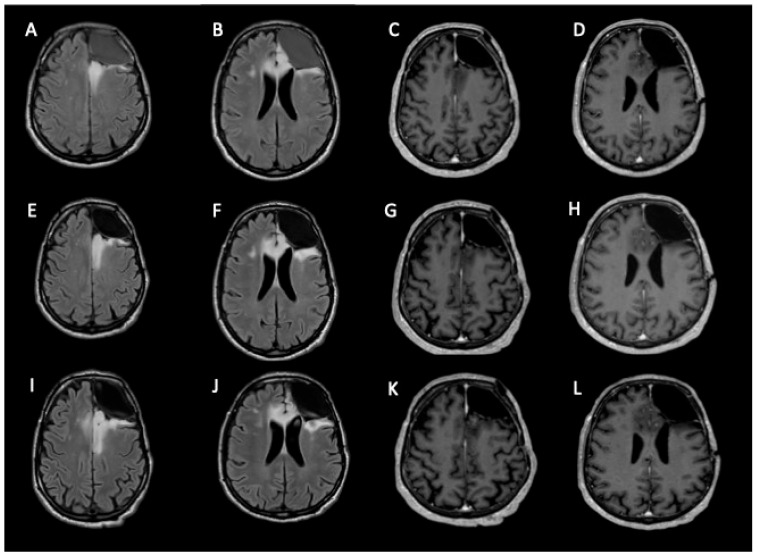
MRI follow-up studies were performed at 4, 10 and 16 months after surgery. The images shown are MRI FLAIR (A,B,E,F,I,J) and post-contrast T1-weighted images (C,D,G,H,K,L). There is progression and infiltration of the residual tumor through the genu of the corpus callosum, involving the left frontomesial cortico-subcortical region and ipsilateral cingulate gyrus, with extension to the contralateral frontomesial region and cingulate gyrus. Subtle areas of contrast enhancement are observed in the left frontomesial region and ipsilateral cingulate gyrus (G,H,K,L).
